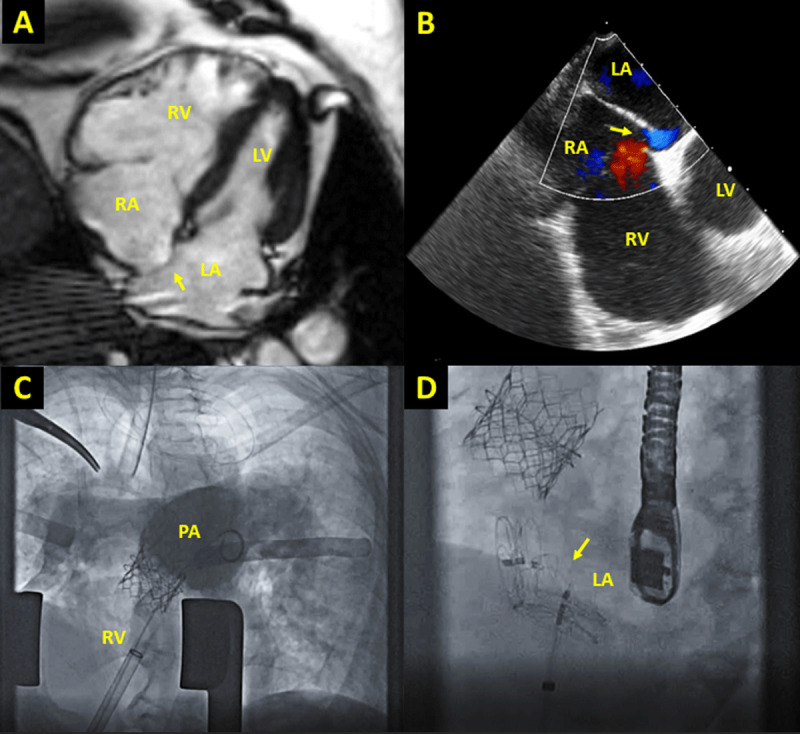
Case imaging. (A) Four-chamber cardiac magnetic resonance imaging demonstrating severely dilated RV compared to LV with leftward bowing of interatrial septum (arrow). (B) Transesophageal echocardiography demonstrating right-to-left shunt through PFO (arrow). (C) Fluoroscopic images from hybrid pulmonary valve replacement and PFO closure; angiogram demonstrates no residual pulmonic regurgitation. (D) Two occluders (arrow) were deployed to close the PFO and atrial septal deflect. LA: left atrium; LV: left ventricle; PA: pulmonary artery; PFO: patent foramen ovale; RA: right atrium; RV: right ventricle.
